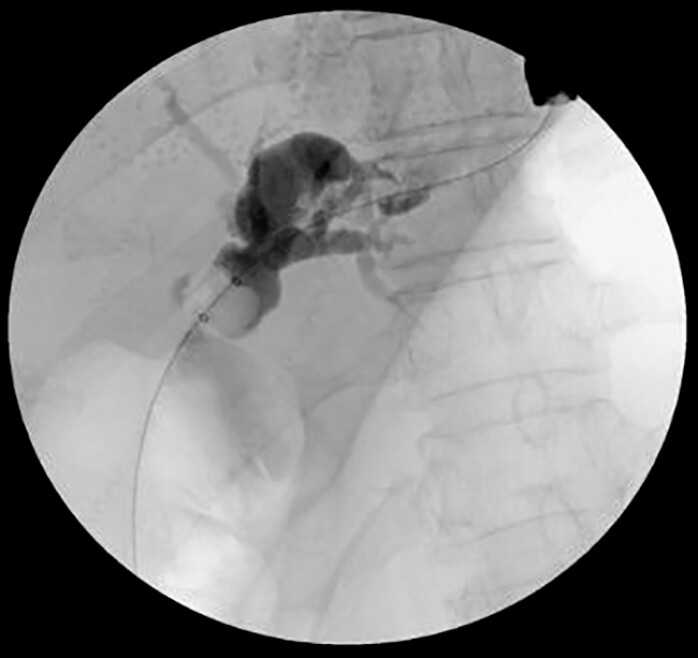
EUS-guided left hepaticogastrostomy for left intrahepatic biliary drainage. EUS, endoscopic ultrasound.
